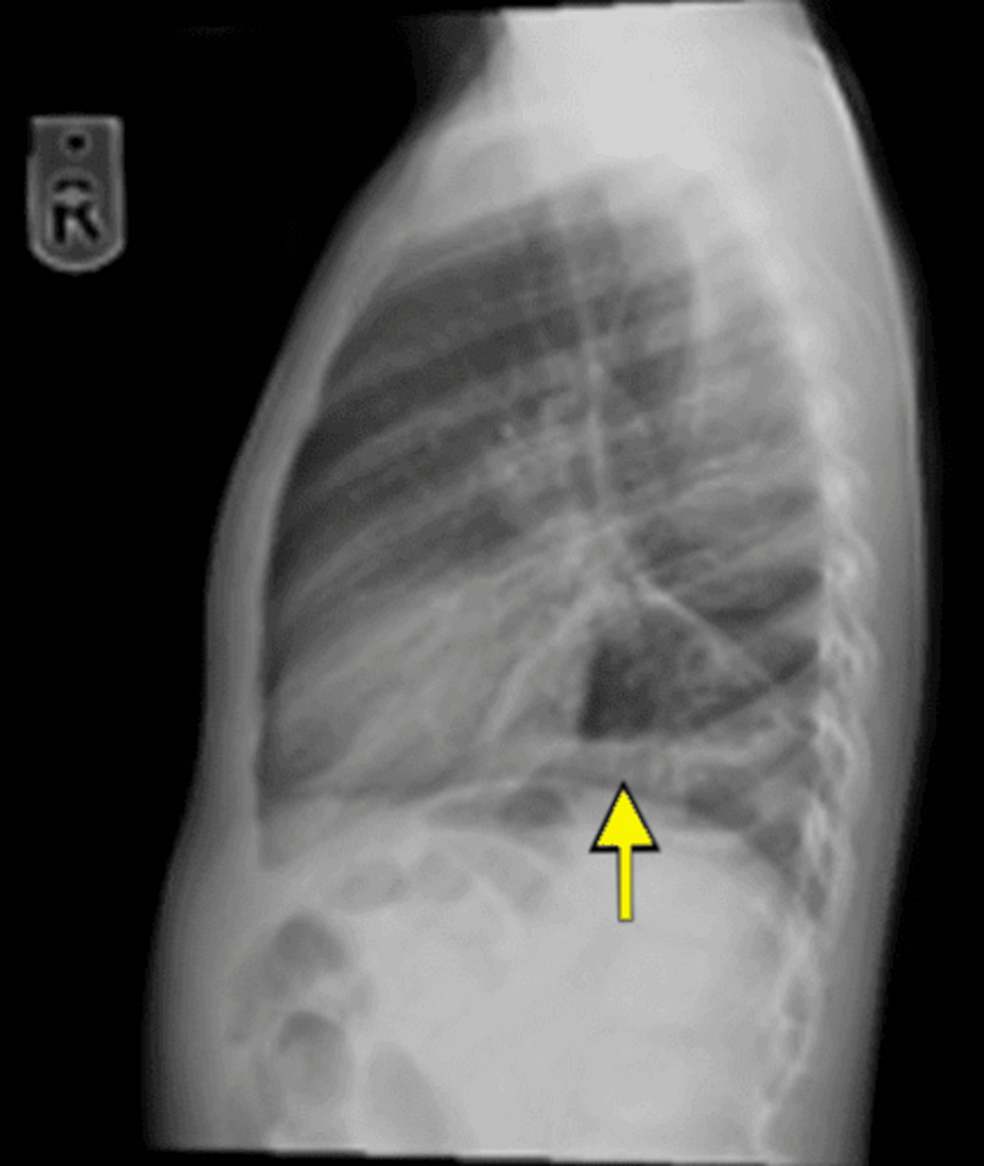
Retrocardiac lucent tubular shadows with air-fluid level; chest CT was then performed to rule out diaphragmatic hernia
